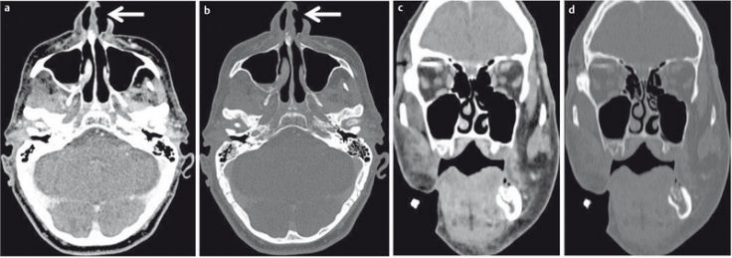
67-year-old patient after surgical treatment of a malignant melanoma of the left nose. CT of the midface in axial (a,b) and coronal (c,d) planes with different window-level settings (soft tissue window level: a,c; bone window level: b,d). The window-level setting has to be chosen according to the tissue to be evaluated.
